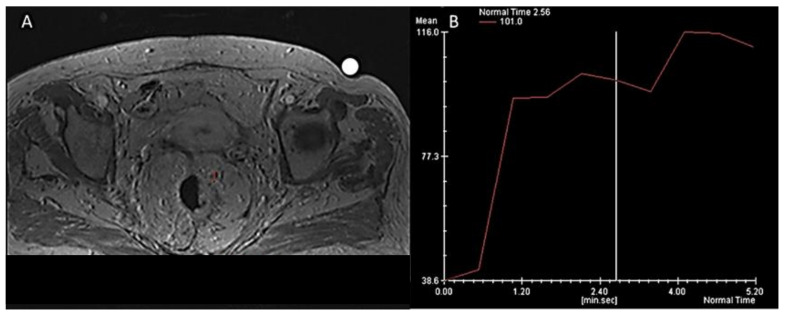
Woman 65 y.o. with rectal cancer (same patient of Figure 2 and Figure 4). DCE- MRI (A) deposit assessment with Intensity/Time curve evaluation (B).
